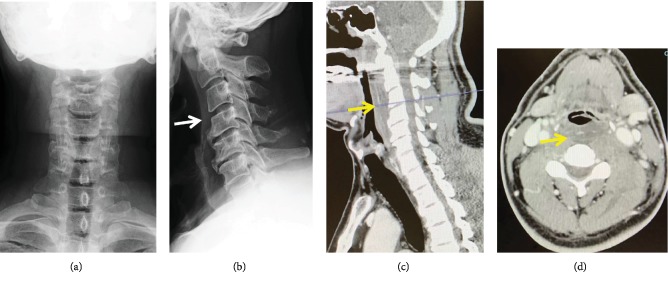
Initial radiographic and computed tomography (CT) assessment of the cervical spine. Posteroanterior (a) and lateral plane (b) radiograms. A lateral plane radiogram showed soft tissue swelling anterior to the vertebral bodies (white arrow). Sagittal (c) and axial (d) CT indicated soft tissue swelling in the posterior pharynx (yellow arrows), but there were no clear signs of abscess formation.
